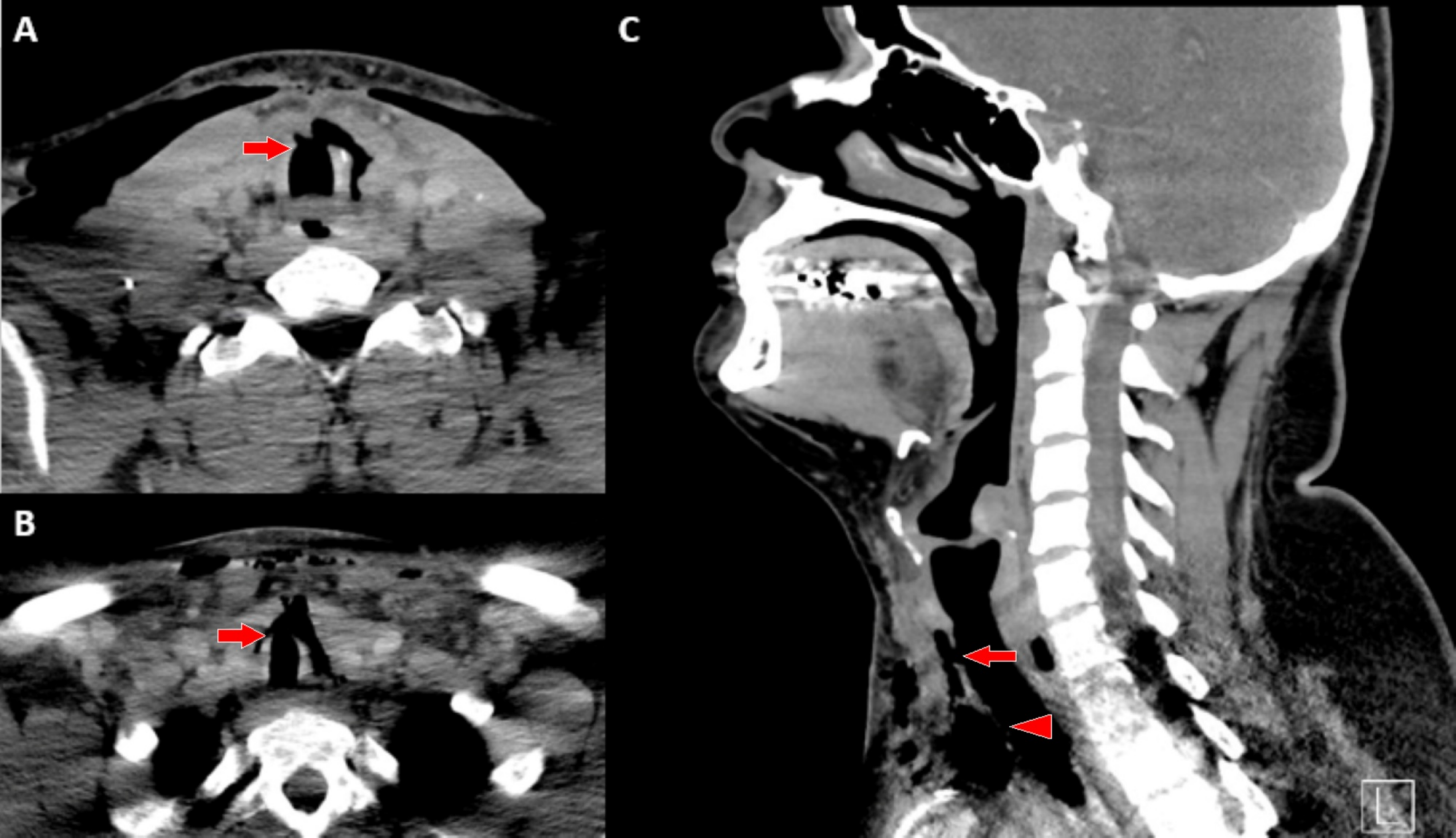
CT of the patient presenting with delayed tracheal injury CT of the neck without contrast demonstrating anterior cervical and mid-tracheal defects with extensive mediastinal and subcutaneous emphysema. (A) Axial cut of anterior cervical defect at rings 2 and 3 (arrow). (B) Axial cut of mid-tracheal defects spanning tracheal rings 9 through 11 (arrow). (C) Sagittal cut demonstrating both anterior cervical (arrow) and mid-tracheal (arrowhead) defects. CT, computed tomography
